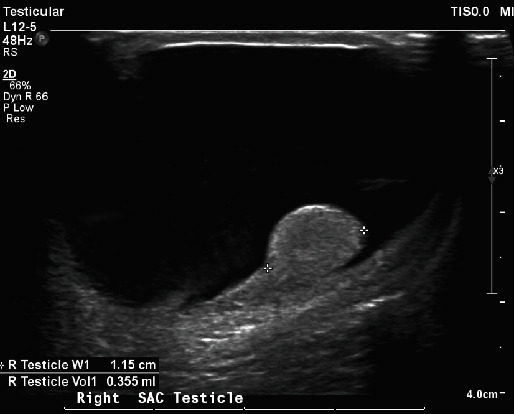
Ultrasound at birth showing large fluid accumulation around a normal right testis.
